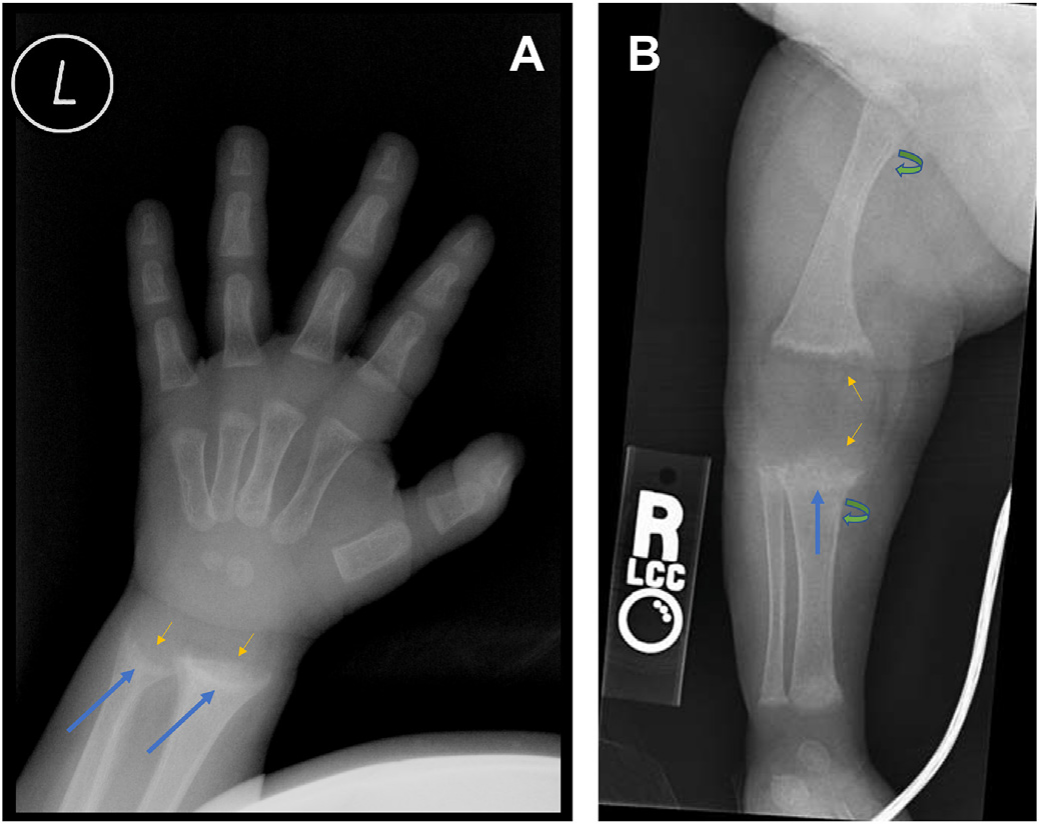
The features are consistent with rickets. In the growing skeleton, deficiency of normal mineralization of the provisional zone of calcification results in fraying and indistinct margins of the metaphysis (short thin yellow arrow), splaying and widening of the metaphyseal ends with cupping or concavity of the metaphysis (long blue arrows) seen in X-rays of both the hand (A) and the knee joint (B). These features are most prominent in the wrist and knee and are also seen at anterior rib ends where they are described as rachitic rosary (not shown). Diffuse periosteal reaction may also be seen along the distal radius and ulna, distal femur, and proximal tibia (curved arrows). Mild bowing of the femur and tibia can occur and is seen in the radiographs (Fig. A: Case courtesy of Dr. Hani Makky Al Salam, Radiopaedia.org, rID: 8971 https://radiopaedia.org/cases/8971 and Fig. B: Case courtesy of The Radswiki, Radiopaedia.org, rID: 11889 https://radiopaedia.org/cases/11889).
